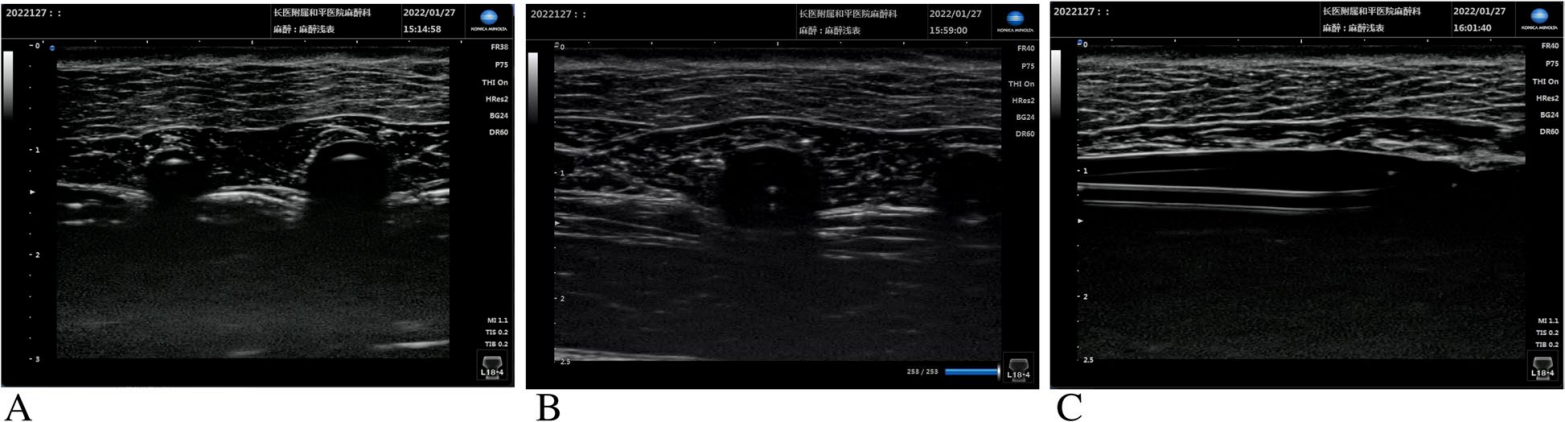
Vascular ultrasound images. when researcher is utilizing a high-frequency ultrasound probe scan the model. A Vessels (left for artery and right for vein). B Confirmation of catheter’s position in the short axis view (sound beam was perpendicular to course of the vessels). C Confirmation of catheter’s position in the long axis view (sound beam paralleled to course of the vessels)
